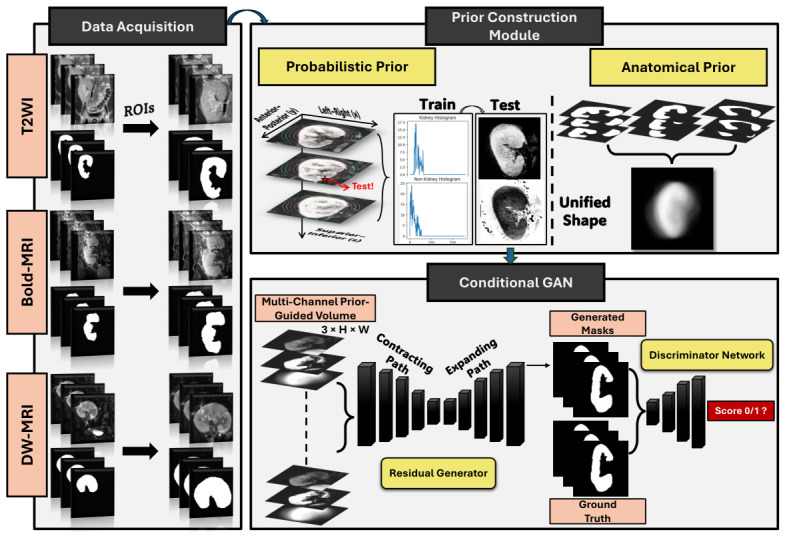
An overview of the proposed prior-guided renal segmentation framework. Each MRI modality is processed independently. First, probabilistic appearance prior and an anatomical shape prior are constructed then concatenated with the ROI image to form a three-channel condition volume that is provided to the conditional GAN to generate the mask volume.
